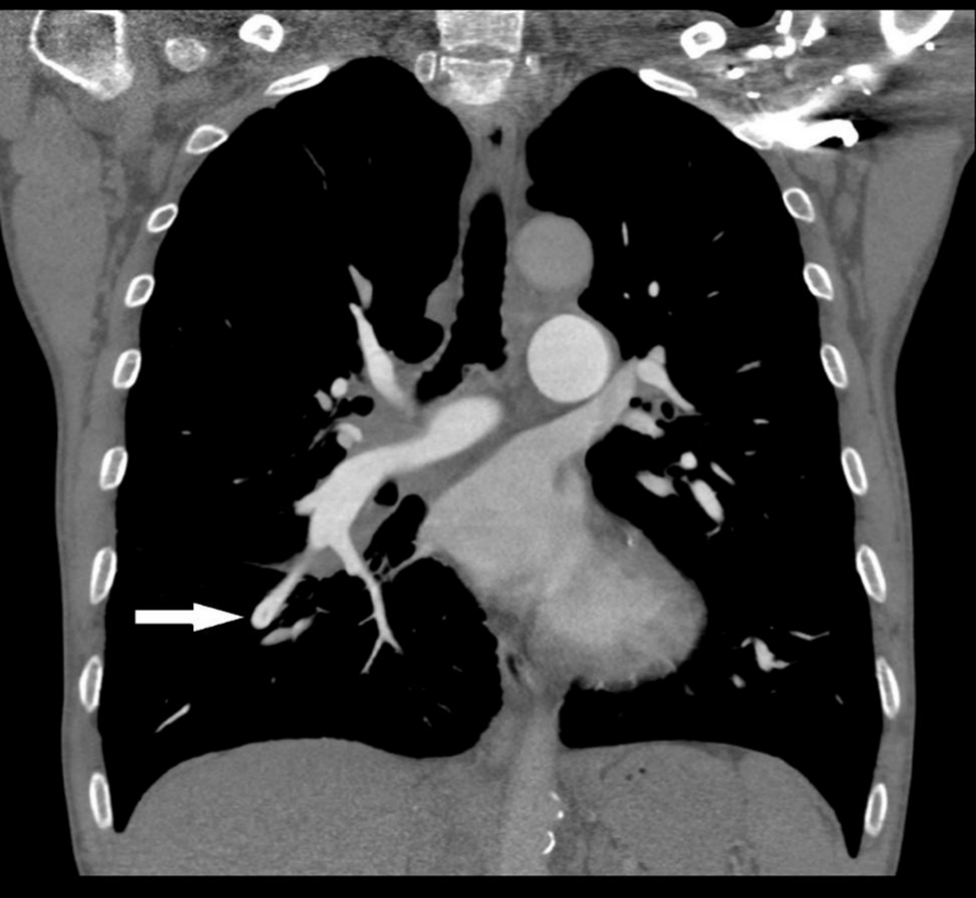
The examination of CT scan.
